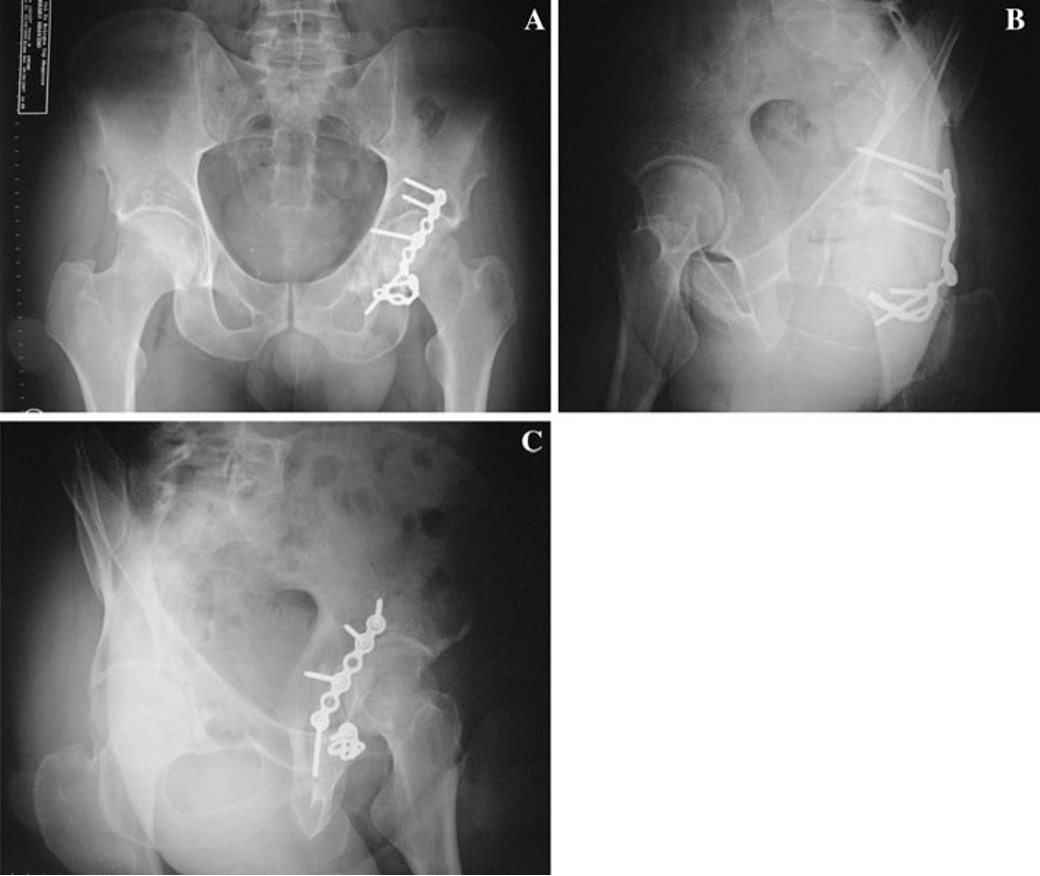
a–c Postoperative X-ray. One plate is on the posterior column to maintain reduction of the posterior wall. The second plate is under the ischium to maintain reduction of the intra-articular fragment and a fragment of the*lamina quadrilatera*
